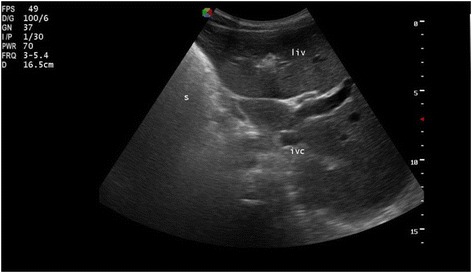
Abdominal ultrasound showing situs inversus (liver and inferior vena cava on left side, and spleen on right side)
